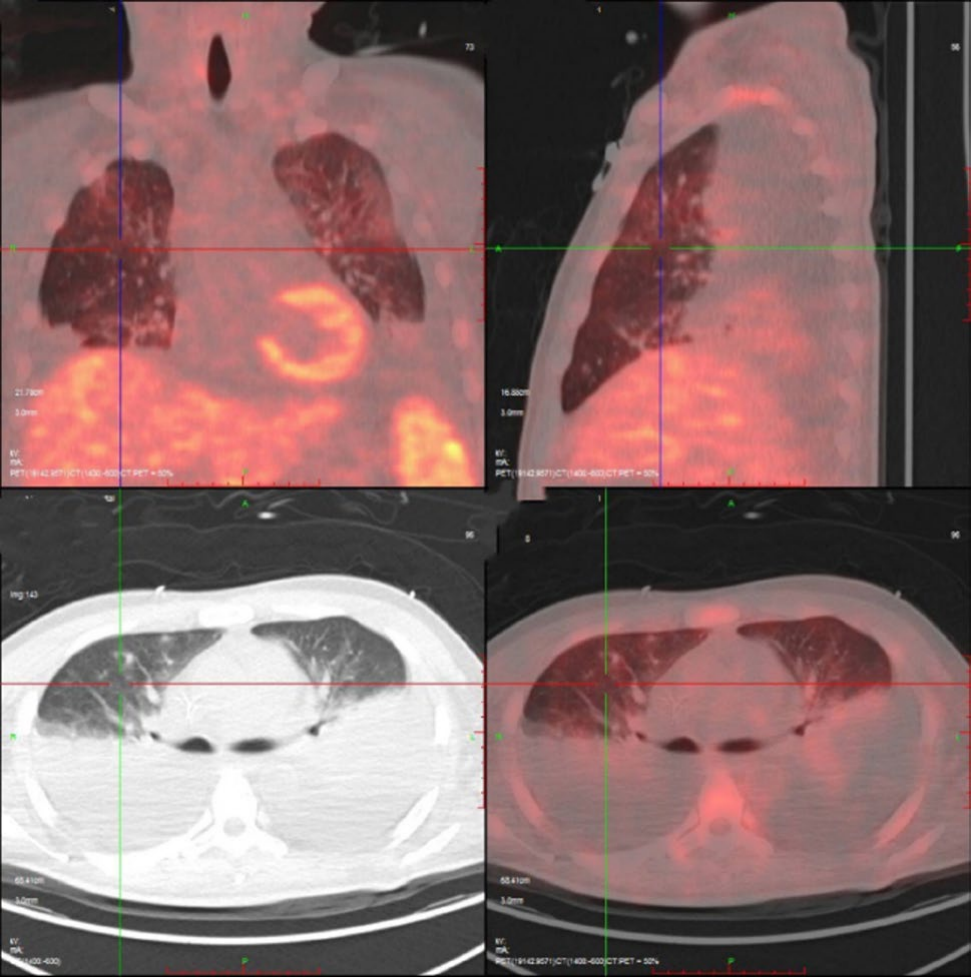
PET-CT imaging reveals bilateral pleural effusions with no abnormal FDG uptake within the pleural fluid. The absence of metabolic activity suggests a reactive effusion secondary to lymphatic obstruction rather than malignant pleural involvement, supporting the diagnosis of chylothorax
